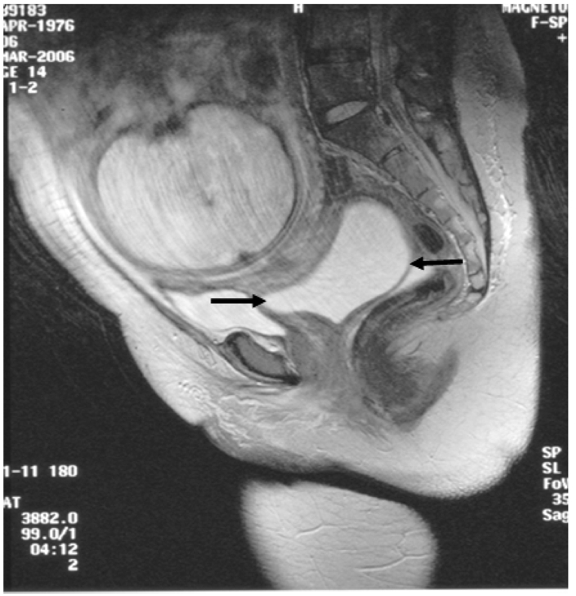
Sagittal T2-weighted MR image of the pelvis shows a cystic mass (arrows) lying posterior to the urinary bladder and inferior to the gravid uterus.
